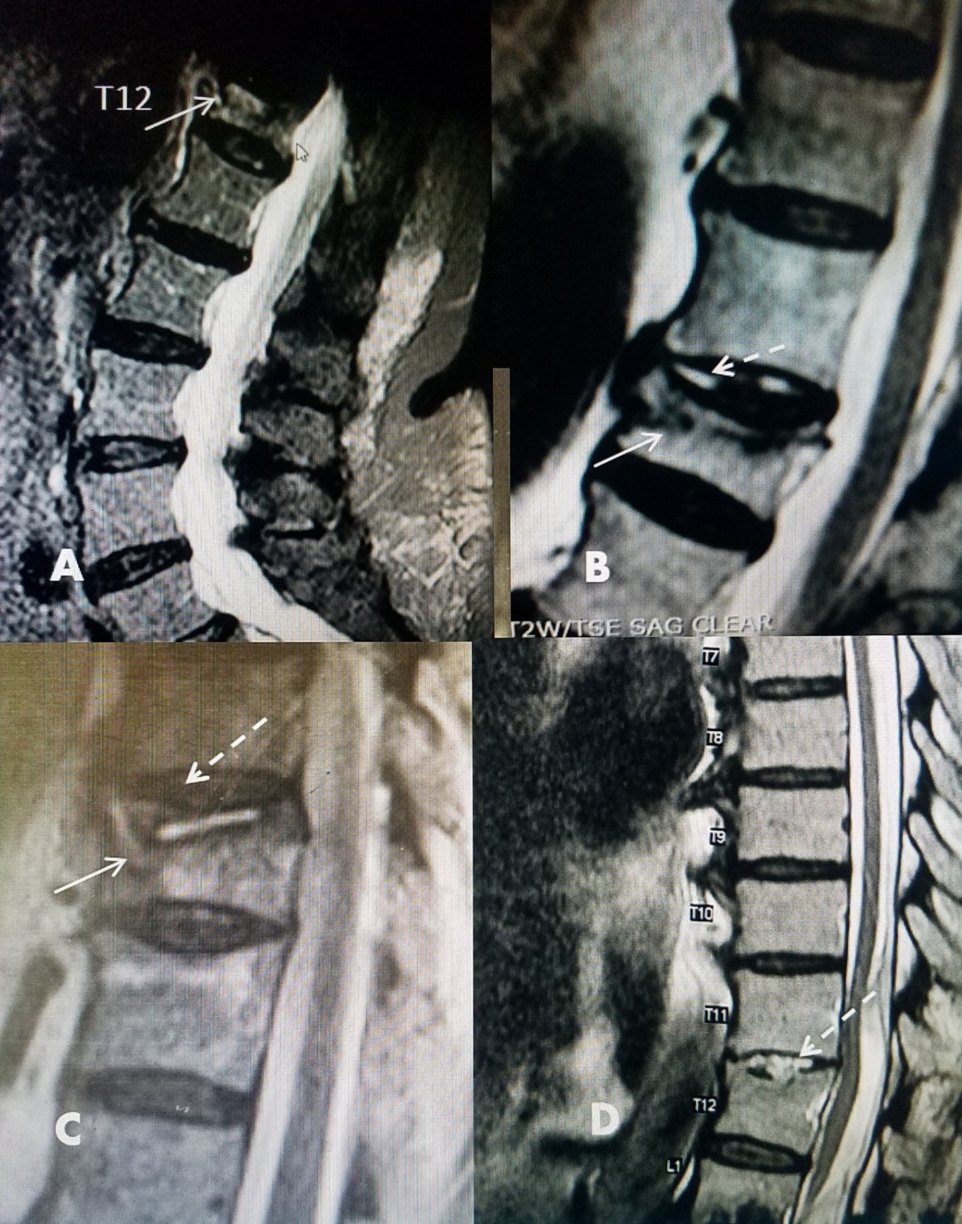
Examples of vertebral clefts with chronic vertebral compression fractures A: Small high-intensity signal cleft (solid white arrow) within the superior collapsed body of T12. B: T2 magnetic resonance imaging (MRI) showing vertebral collapse, edema (solid white arrow), and vertebral cleft in the superior endplate as well as the disc space (dashed white arrow) at L2. There are a broad-based collapse and mild kyphotic angulation. C: Sagittal T2 MRI in a patient with a T11 chronic fracture with an anterior partial vertebral collapse within the body without angulation (solid white arrow). There is a long high-intensity fluid cleft along the middle part of the superior endplate (dashed white arrow). D: T11-T12 high-intensity fluid cleft extending into the disc space just above a small superior endplate fracture in T12 (dashed white arrow). There is slight kyphotic angulation at the T11-T12 level.
